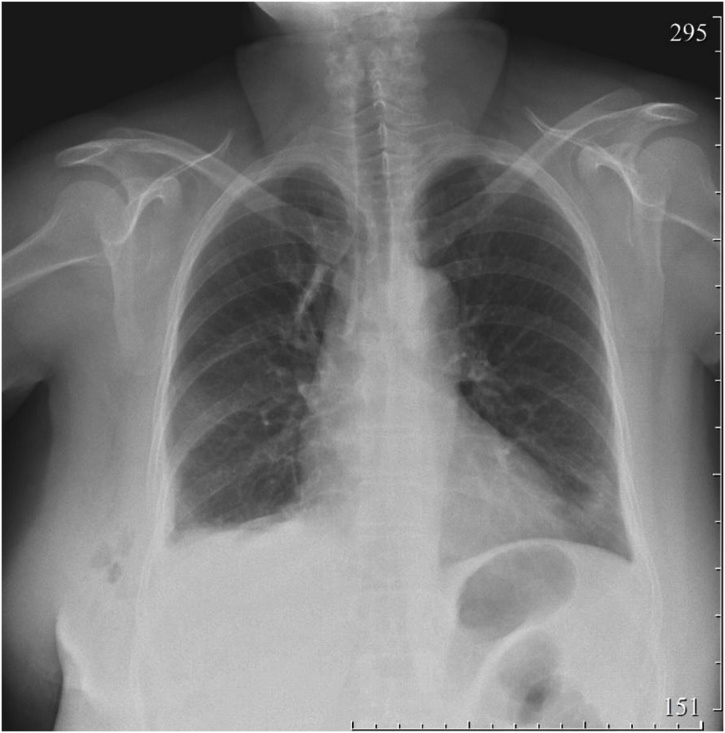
Afteroperative chest X-ray: formed right diaphragmatic dome at the seventh intercostal space.
